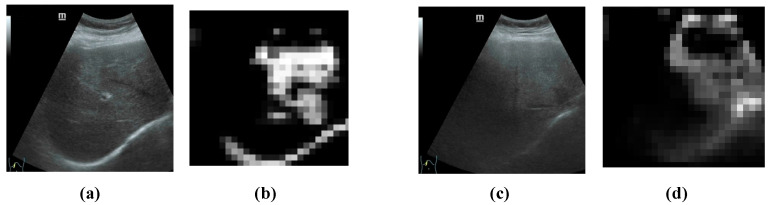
BiNet feature extraction map. From left to right, (a) normal liver ultrasound image, (b) feature map extracted from normal liver ultrasound image, (c) fatty liver patient ultrasound image, and (d) feature map extracted from fatty liver patient ultrasound image.
